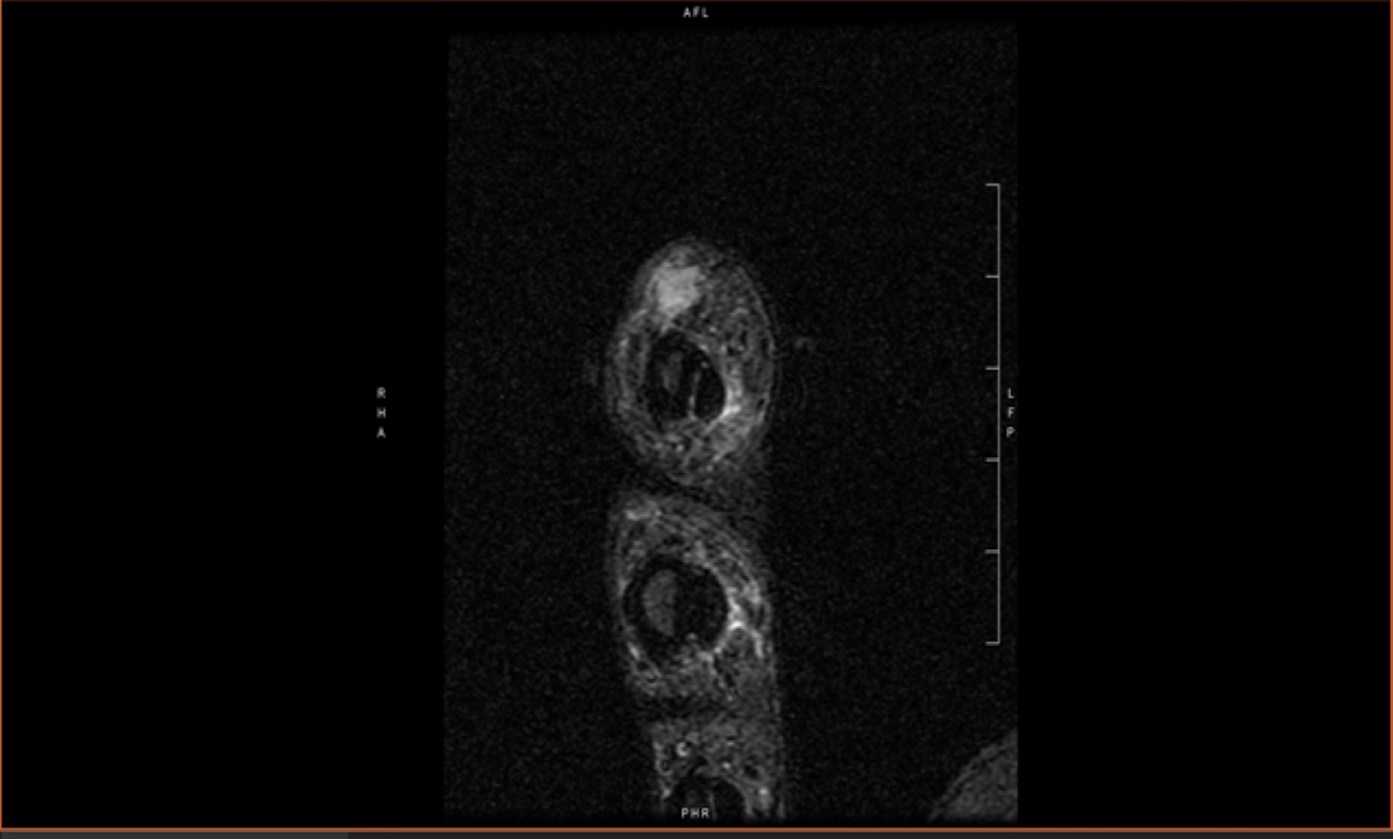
Magnetic resonance imaging of left hand showing approximate 1 cm subcutaneous mass on lateral aspect of index finger.
